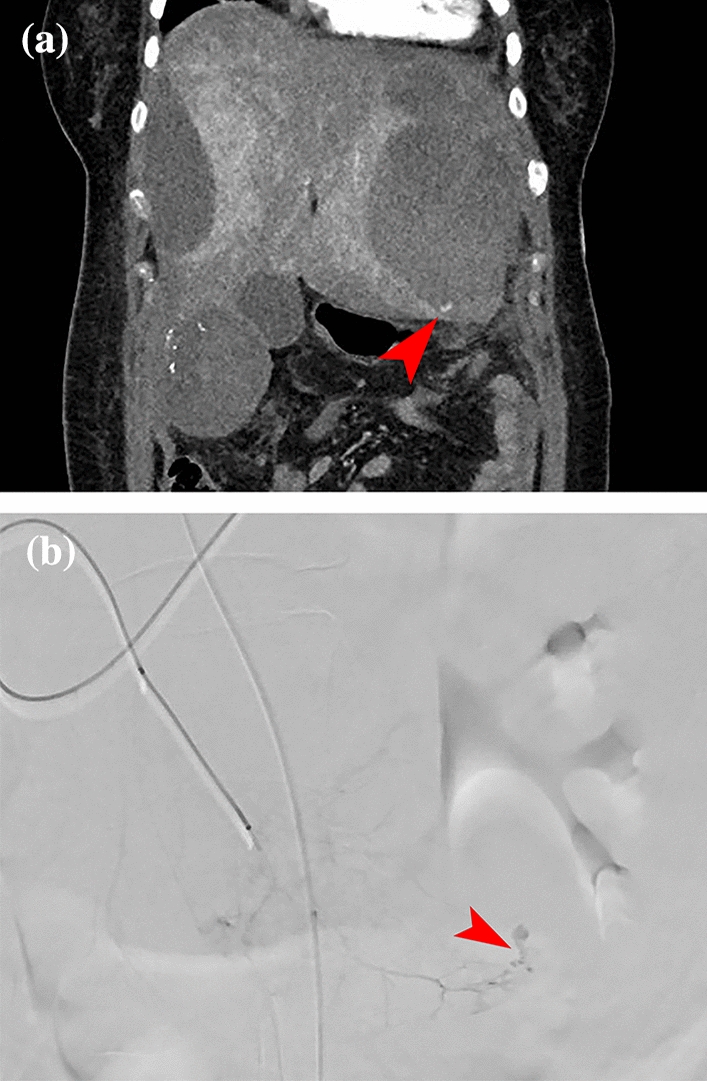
Images following persistent suspicion of recurrent bleeding. A Additional abdominal CT shows a small arterial bleed with concomitant hematoma, breaking through the capsule, resulting in a hematoma in the left paracolic gutter extending into the small pelvis (marked with red arrow). B The bleed as shown during selective catheterization (marked with red arrow)
